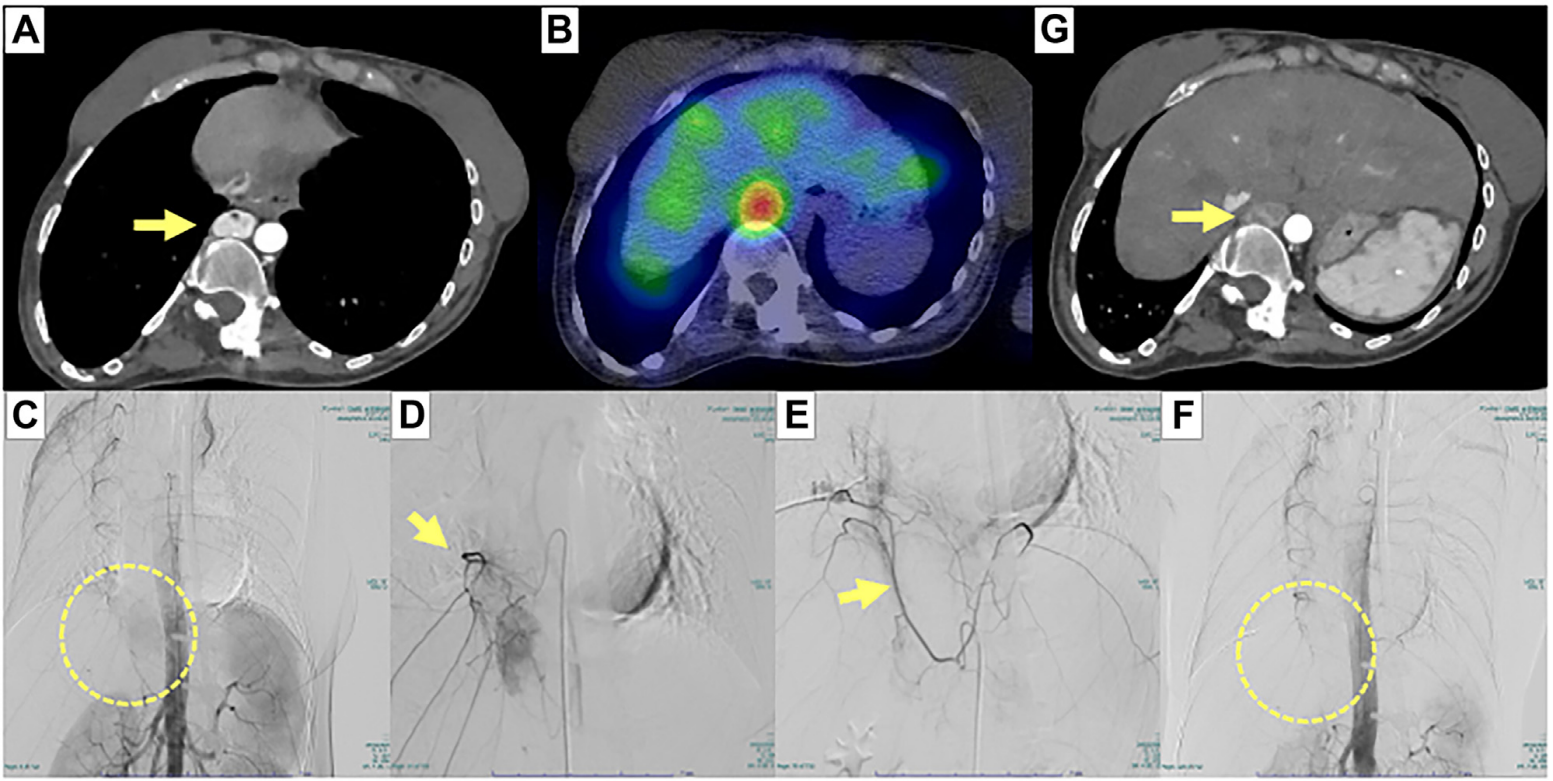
Preoperative embolization of a primary paraganglioma in the mediastinum. (A) Computed tomography (CT) shows paraganglioma primarily in the mediastinum before embolization (yellow arrowhead). (B) ^123^I-metaiodeobenzylguanidine shows abnormal accumulation (maximum standardized uptake value) in the same region. (C) Preoperative embolization is performed for a paraganglioma in the mediastinum. A catheter is inserted into the right 10th intercostal artery, and contrast is imaged (yellow circle). (D) Embolization is performed on the right 10th intercostal artery with embolic material (yellow arrowhead). (E) The right subdiaphragmatic artery is contrasted and embolized with a metal coil and embolic material (yellow arrowhead). (F) The tumor’s loss of contrast is evident after embolizing the tumor’s feeding arteries (yellow circle). (G) CT shows the contrast effect inside the mass is weakened (yellow arrowhead) after the embolization.
